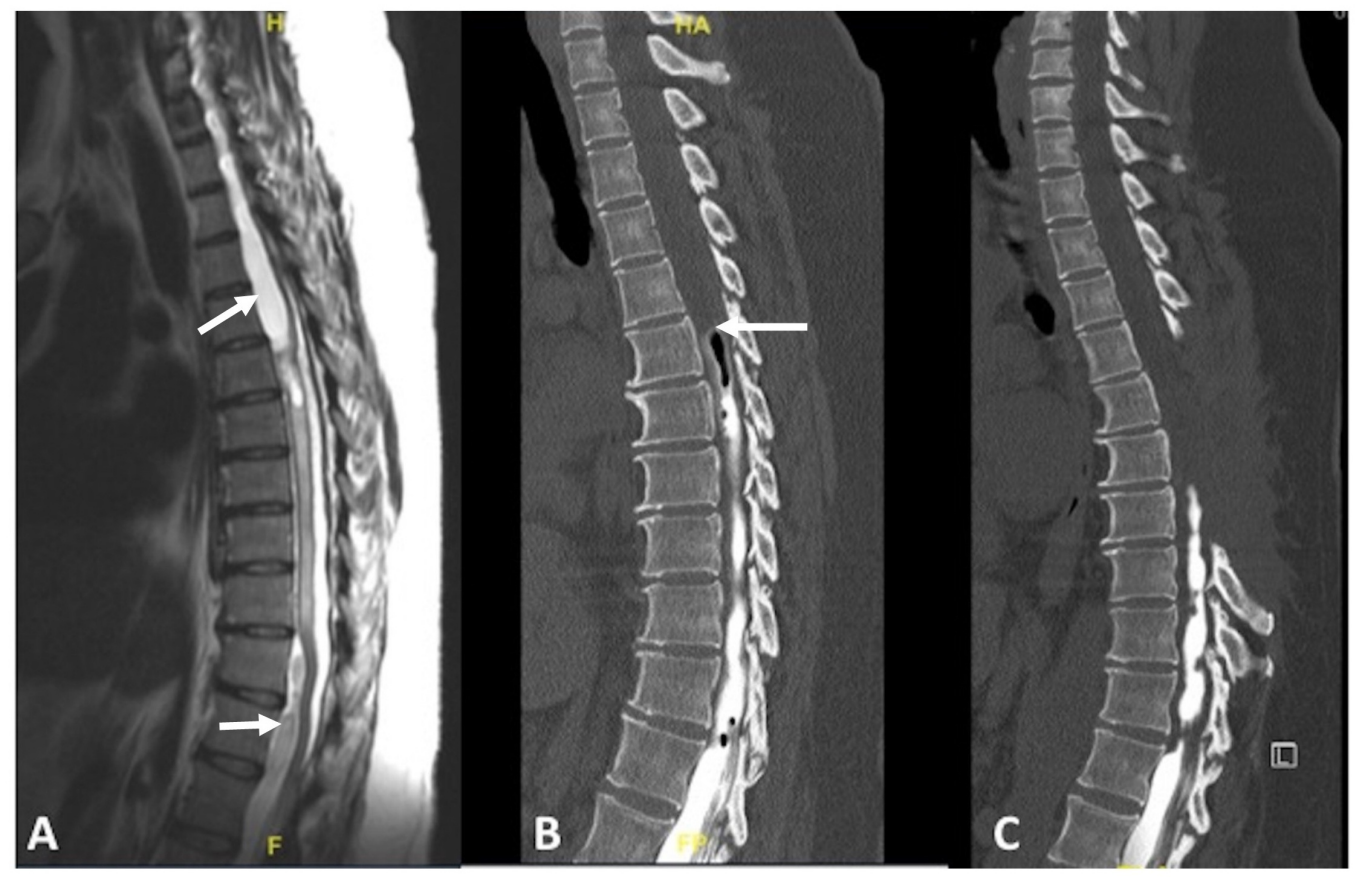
T2-weighted MRI and CT myelogram demonstrating spinal arachnoiditis with associated arachnoid cysts. (A) T2-weighted sagittal MRI without contrast showing arachnoid cysts (arrows) ventral to the thoracic and lumbar spinal cord with cord compression. The spinal cord is ventrally displaced at the T5-8 levels due to arachnoiditis in the dorsal leptomeninges; (B) CT myelogram sagittal view showing stagnation of contrast at the T5 level (arrow), a sign that arachnoiditis is blocking cerebrospinal fluid flow; (C) Postoperative myelogram showing T5-8 laminectomy with no improvement in cerebrospinal fluid flow. MRI: magnetic resonance imaging; CT: computed tomography
